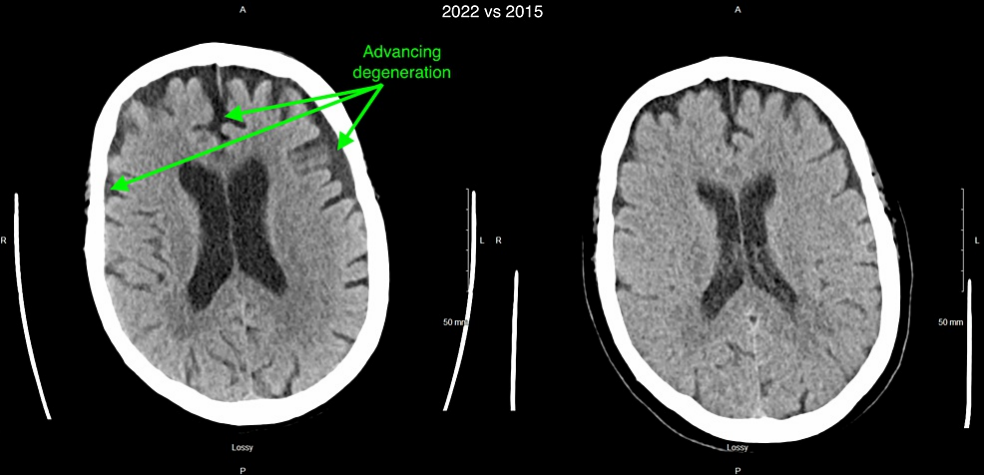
Computed tomography (CT) without contrast of the head 2022 vs 2015 (2) 2022 scan is on the left while 2015 scan is on the right
